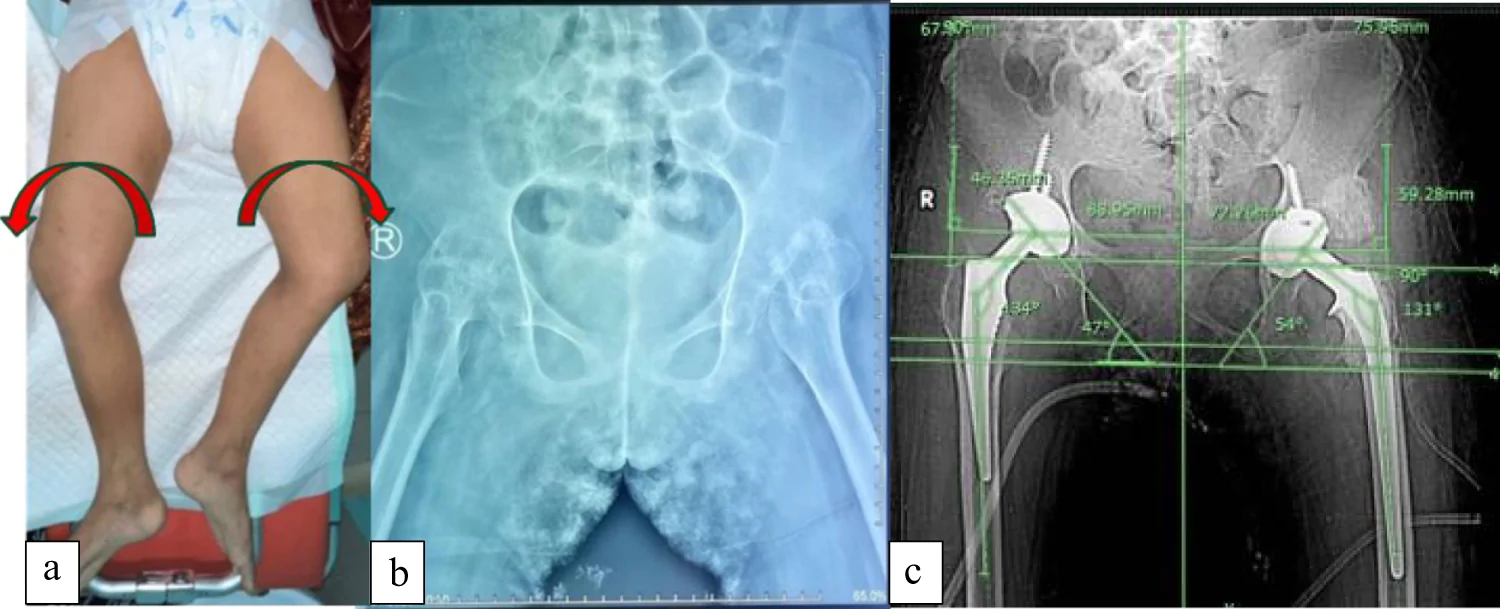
Preoperative and postoperative clinical and radiological images of the hips: (a) clinical photographs of the right and left hips in external rotation; (b) radiographs of the pelvis before surgery; (c) radiographs of the pelvis after surgery.
